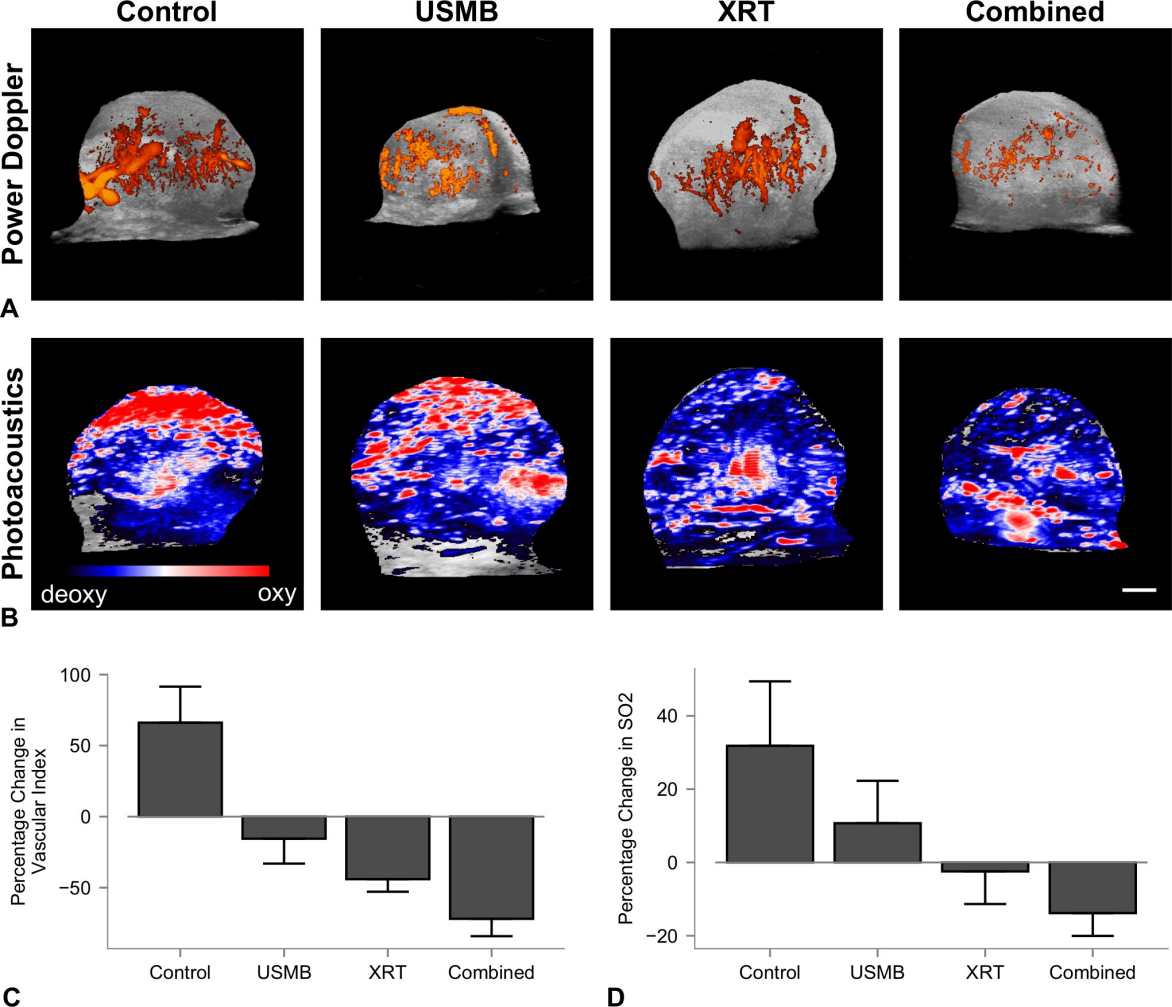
Volumetric 3D power Doppler depicting blood flow and photoacoustic imaging depicting changes in oxygen saturation. (A) Maximum intensity projections of volumetric 3D power Doppler depicting blood flow 24 hours after treatment, (B) and photoacoustic imaging depicting the changes in oxygen saturation. Coloured overlays represent 0–40 dB for power Doppler imaging, and blue-red colour bar represents relative ratio of oxy- and deoxyhemeglobin levels. Columns represent untreated tumors, 1% (v/v) microbubble and focused ultrasound exposure (USMB), 8 Gy radiation exposure (XRT), and combined treatments. The scale bar represents 4 mm. (C and D) Quantified percentage change in VI and oxygen saturation. Error bars represent SEM.
